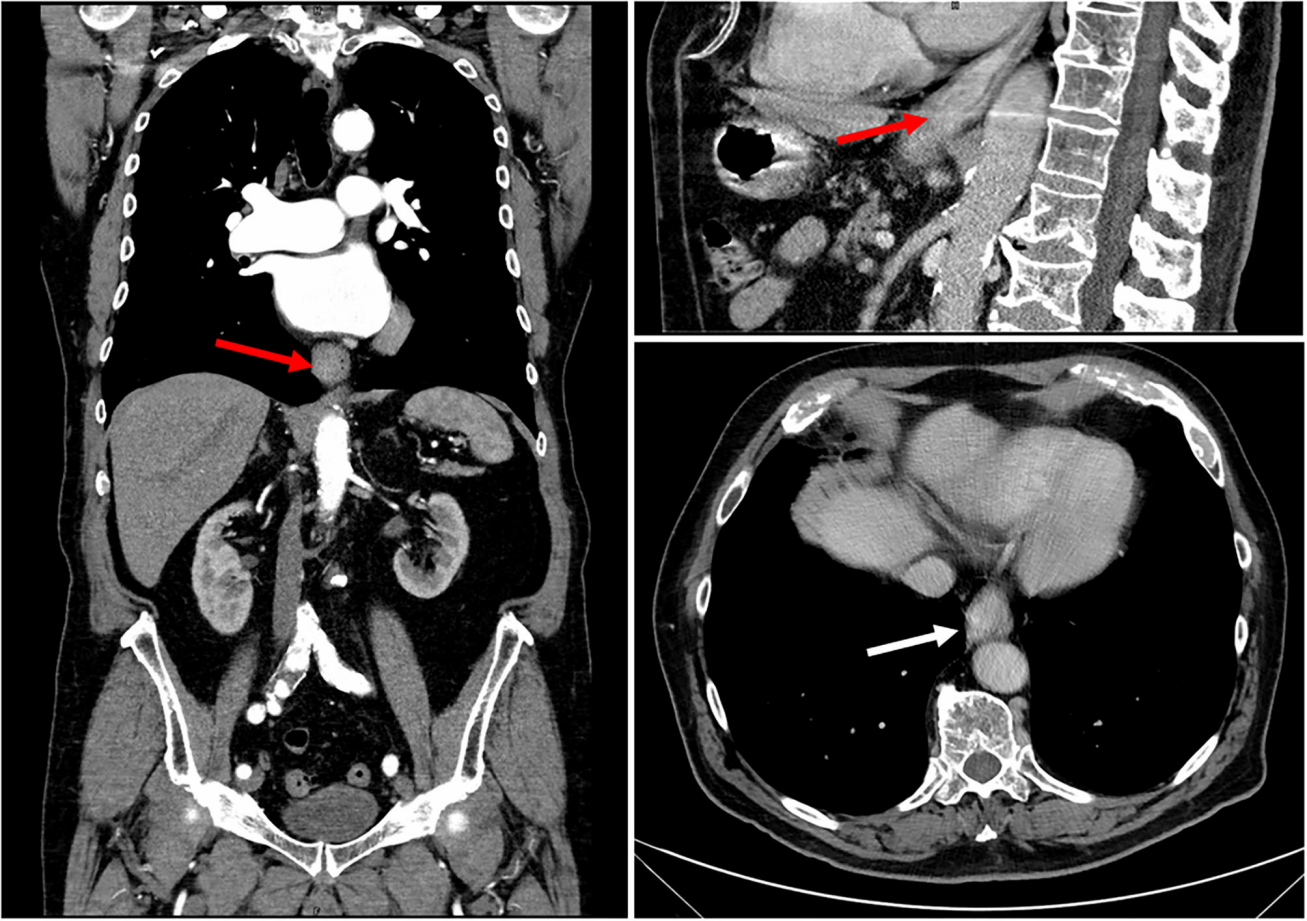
CT scan of the esophageal mass showing asymmetrical neoplastic wall thickening, extending from the lower third of esophagus to the cardia (white arrow in axial view and red arrows in sagittal and coronal views). The mass caused esophageal stenosis without dilatation of the upstream lumen.
